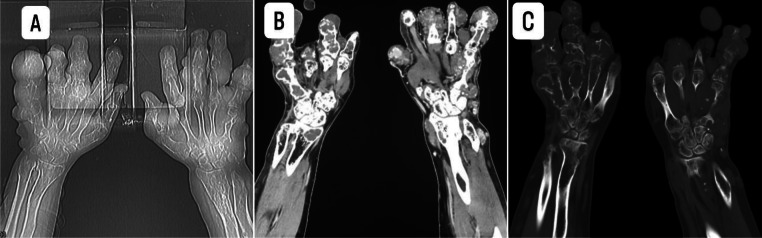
The scout X-ray (A) reveals extensive bilateral bony destruction involving the metacarpals, phalanges, and distal radii. There is irregular deformity and deviation of the distal radius, more pronounced on the right side, along with multiple expansile destructive bone lesions, consistent with enchondromas. The soft tissue window (B) demonstrates the presence of soft tissue components associated with the bony lesions. Additionally, multiple hemangiomas are noted within the surrounding soft tissues. The bone window (C) highlights extensive intramedullary expansile destructive lesions involving the metacarpals, phalanges, and distal radii/ulnae bilaterally. There is significant cortical thinning, with multifocal areas of cortical breakthrough, further supporting the diagnosis of multiple enchondromas with possible malignant transformation.
